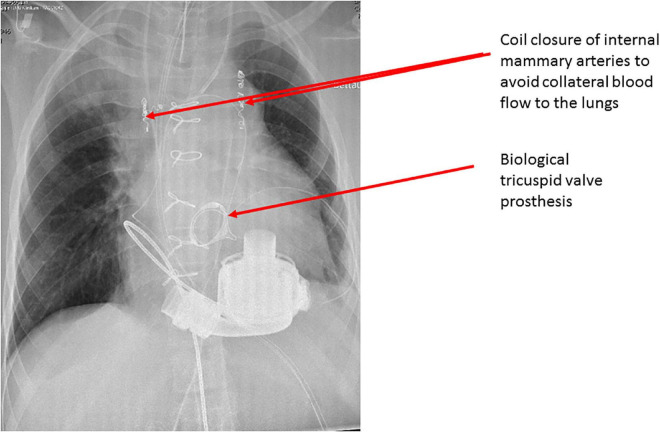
Postoperative chest x-ray showing adequate positioning of the Heart Mate 3 device in the right ventricle as well as the tricuspid valve bioprosthesis.
